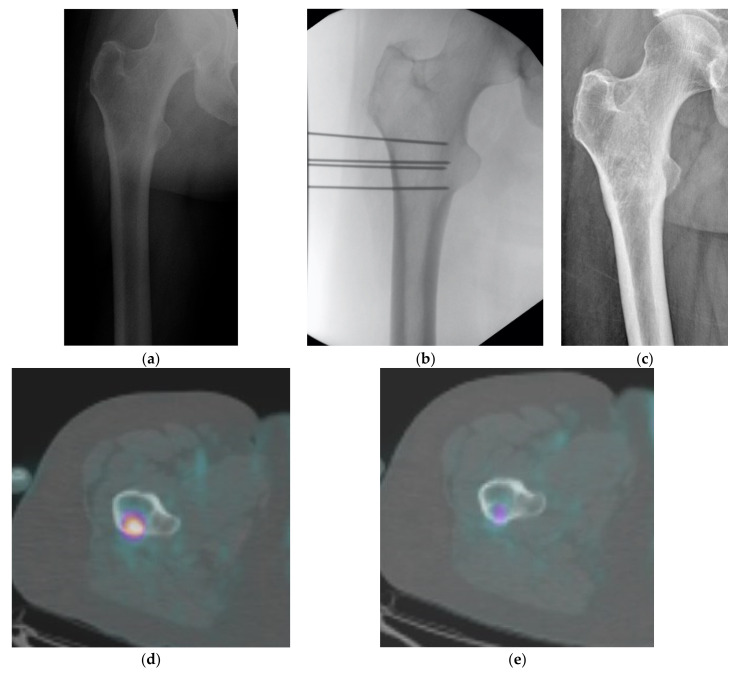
(a–e) Woman 47 years old. Osteolytic lesions in proximal femur in bone metastasis from breast carcinoma treated with ECT. (a) Pre-op X-ray, (b) intraoperative fluoroscopic check, (c) 18 months of follow-up: pain decreased from VNS 9 to 0/10 and the lesion partially ossified. PET scan performed before (d) and 6 months after treatment (e) shows a partial metabolic response of the lesion.
